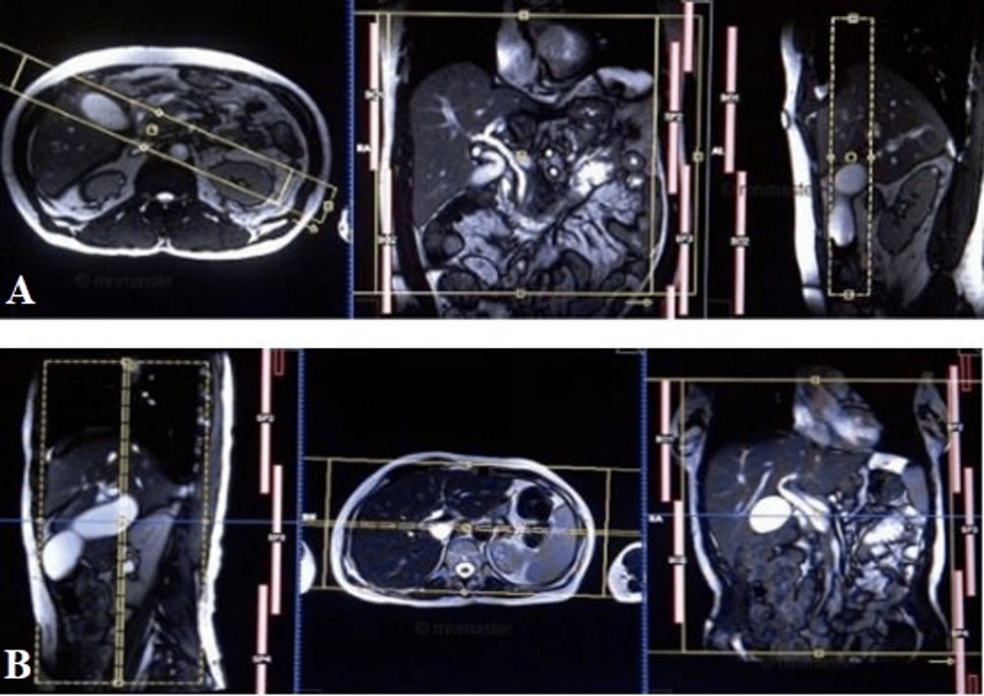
Optimizing T2-weighted MRCP imaging: Axial and coronal planning strategies (A) To plan the T2-weighted axial section of the gallbladder (GB) with a yellow dashed box in MRCP, ensure the imaging slice is positioned parallel to the GB's long axis for comprehensive visualization. Adjust slice thickness and orientation to optimize clarity of the GB and surrounding structures, including the common bile duct and the liver. (B) To plan the T2-weighted coronal section of the gallbladder (GB) with a yellow dashed box in MRCP, ensure the imaging slice is positioned perpendicular to the GB's long axis for comprehensive visualization. Adjust slice thickness and orientation to optimize clarity of the GB and surrounding structures, including the common bile duct and the liver.
